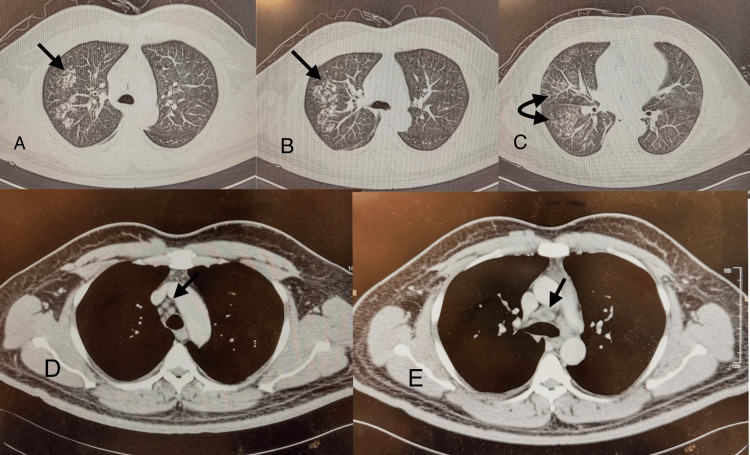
Contrast-enhanced CT thorax before treatment (A, B, and C) Contrast-enhanced CT thorax before treatment. Axial CT images in the lung window demonstrate patchy consolidation seen in the right upper, middle, and lower lobes (straight black arrows in A and B), with a tree-in-bud lesion and ground-glass opacities highlighted by curved black arrows in (C). (D and E) Axial CT images in mediastinal window reveal enlarged mediastinal lymph nodes. The straight black arrow in (D) indicates enlarged nodes seen in the pre-vascular and paratracheal regions, and the straight black arrow in (E) highlights an enlarged node in the pre-carinal region. CT: computed tomography
